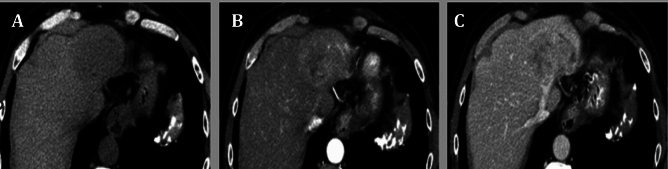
Triphasic CT images showed Large left lobe hepatic focal lesion (A) showed arterial enhancement (B) with rapid washout in delayed phase (C).
